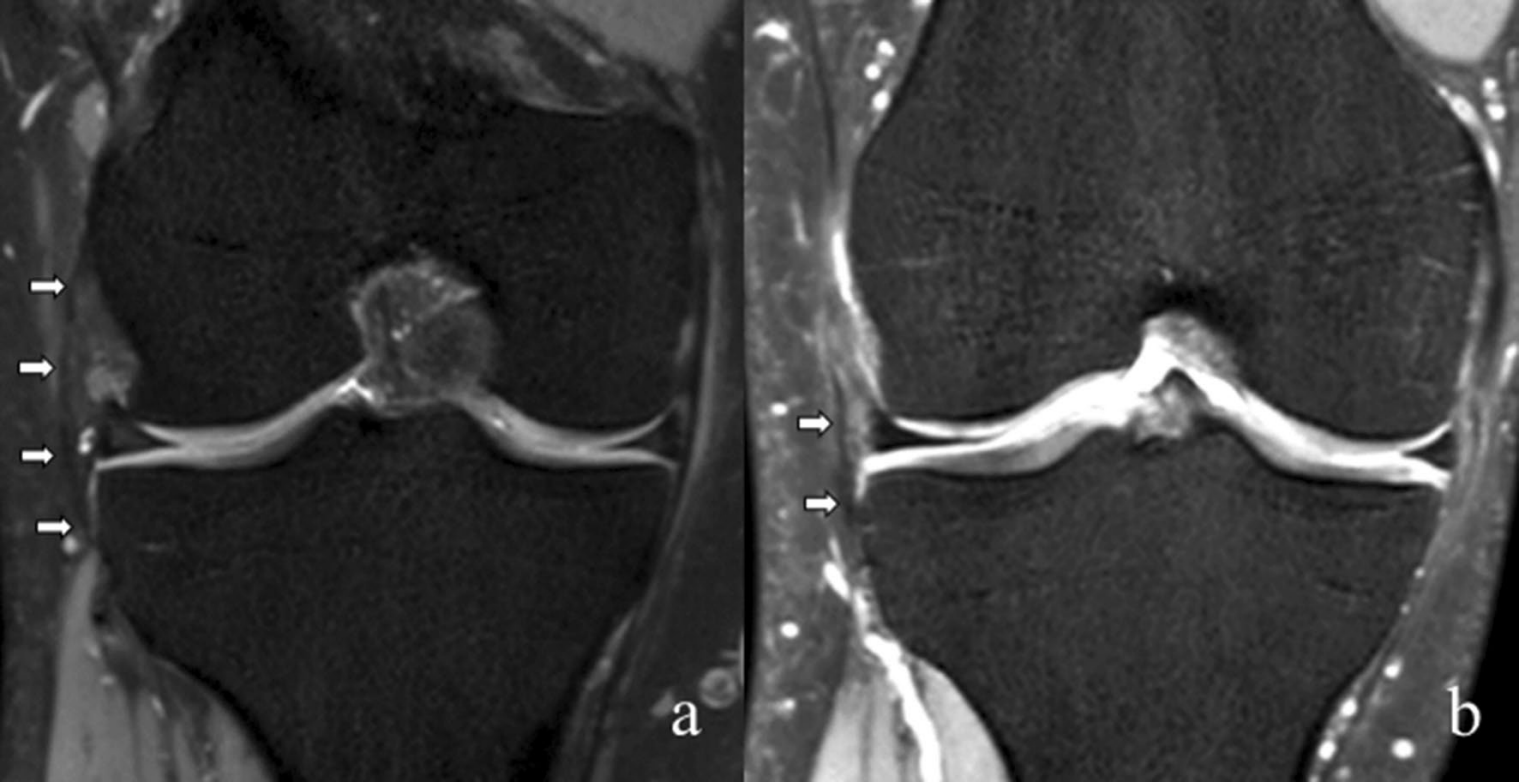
Standard coronal magnetic resonance images of right knees with the anterolateral ligament (ALL) (bold arrows) completely visible (a) with femoral and tibial insertion as well as the meniscal portion. The right image shows a partially visible (b) ALL.
